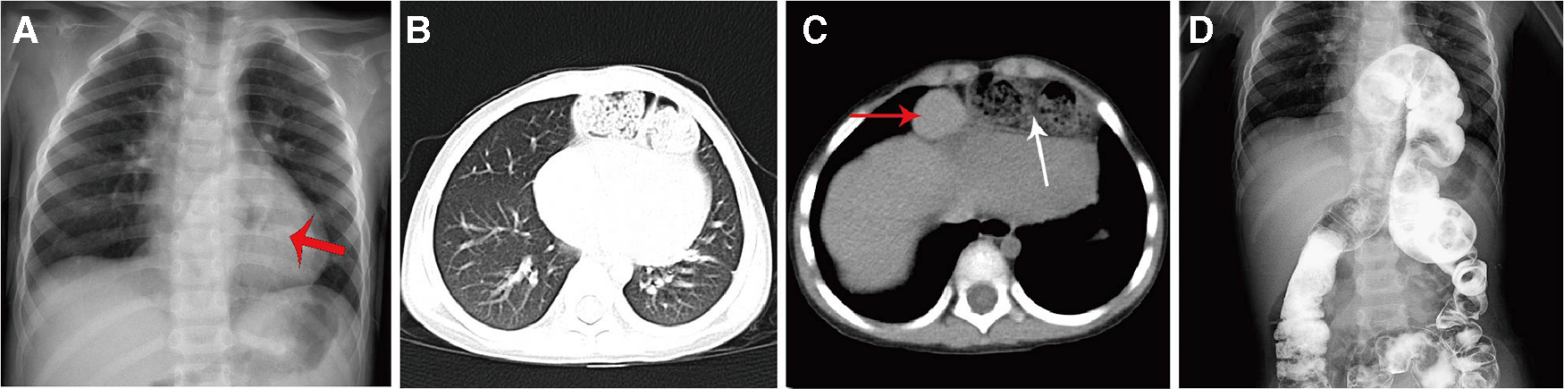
Preoperative imaging of a patient with a bilateral CMH. (A) Routine chest x-ray showing a left sided pericardial mediastinal shadow (red arrow); (B) Chest CT showing a shadow behind the sternum; (C) Chest CT showing partial herniation of the left lobe of the liver into the thoracic cavity, and herniation of the intestine into the thoracic cavity; (D) Barium enema showing herniation of the transverse colon above the diaphragm into the thoracic cavity.
